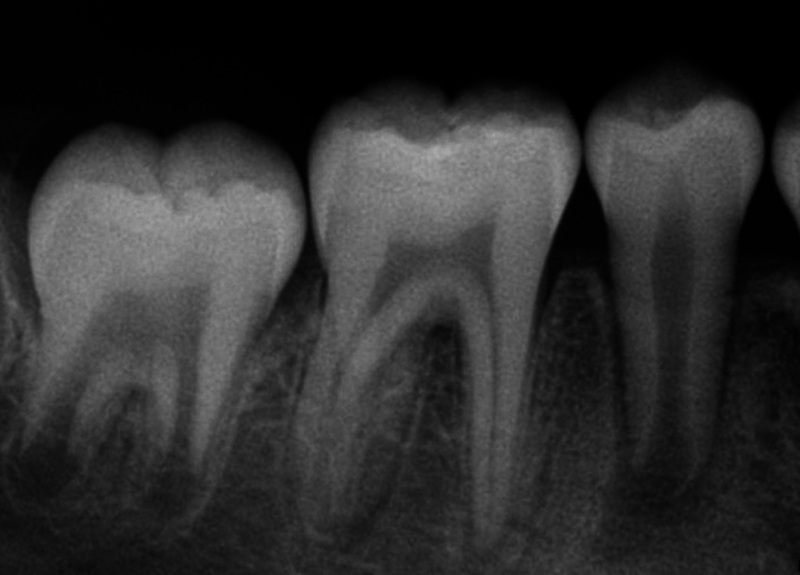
Preoperative periapical radiograph of tooth #29 taken from referring provider.
